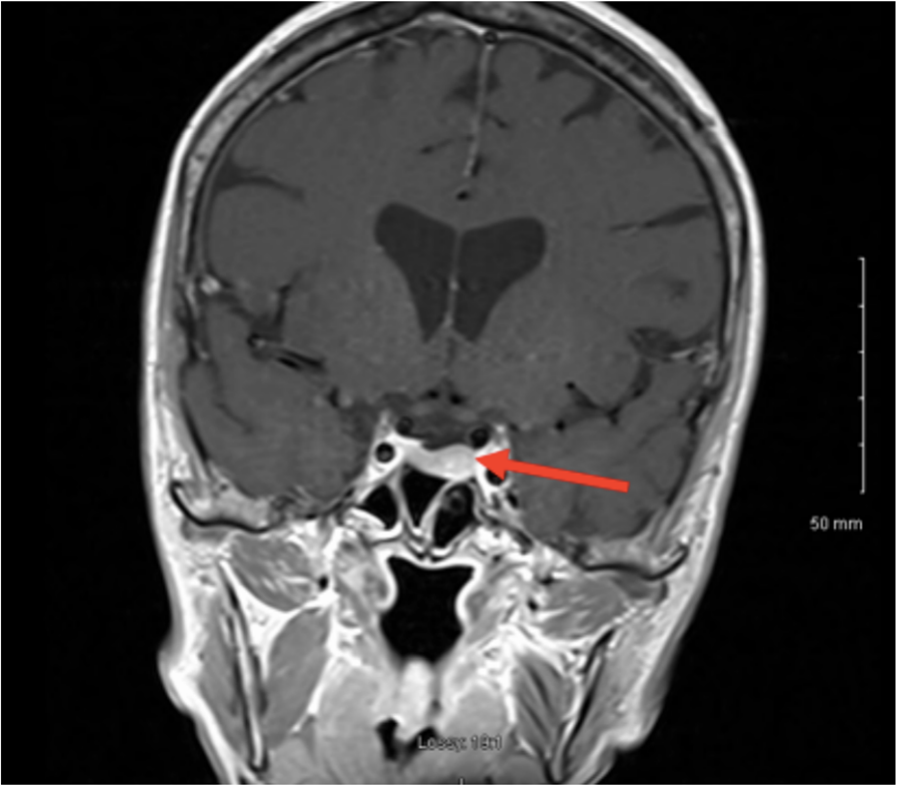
MRI Pituitary. MRI Pituitary showing enlargement of the left side of the pituitary - findings suspicious for a pituitary microadenoma
